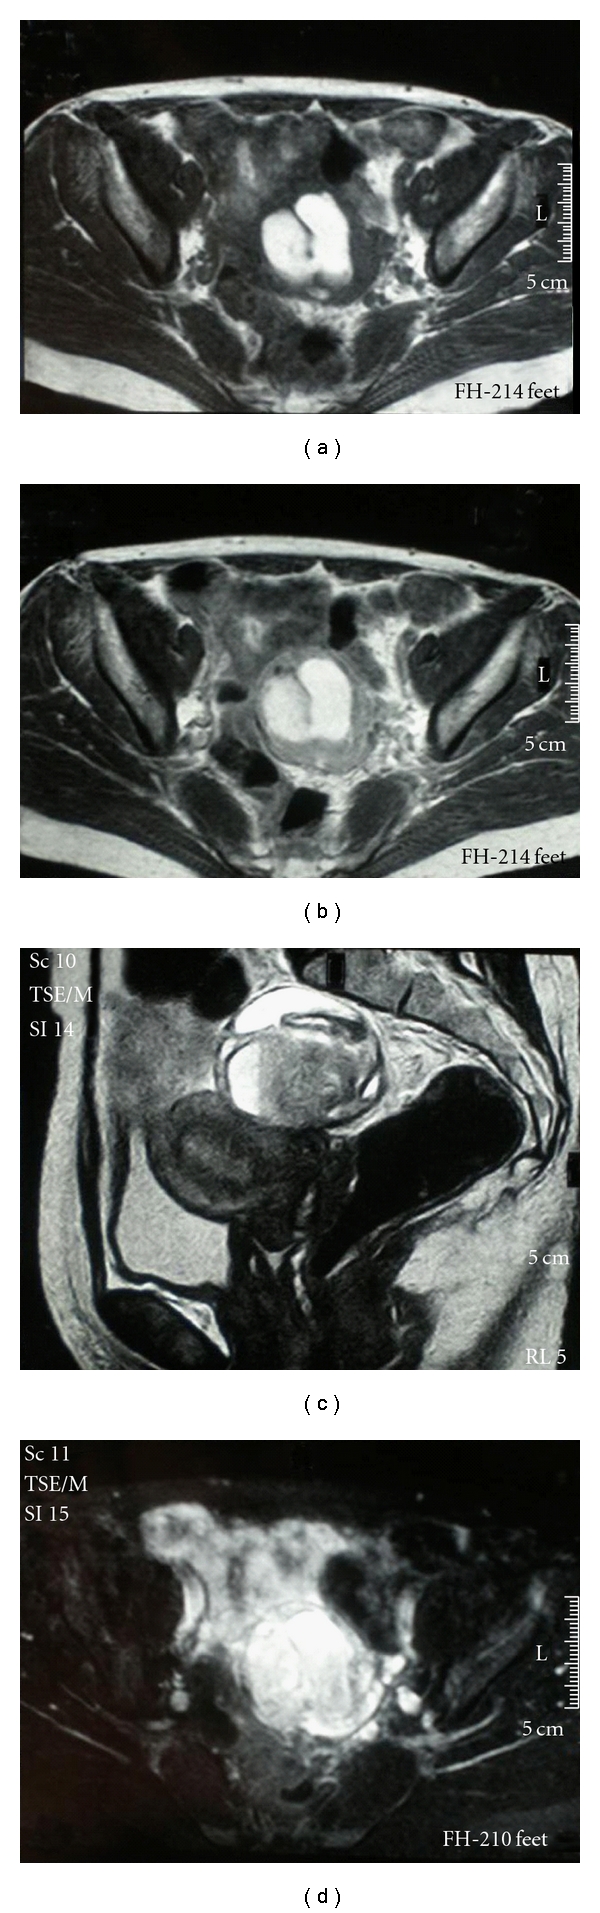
(a-b) T1-weighted MR image shows a unilocular high-signalintensity mass on the left side. (c-d) T2-weighted image shows that the left-sided mass remains high in signal intensity. The variable appearance of the lesion is thought to reflect the blood products.
